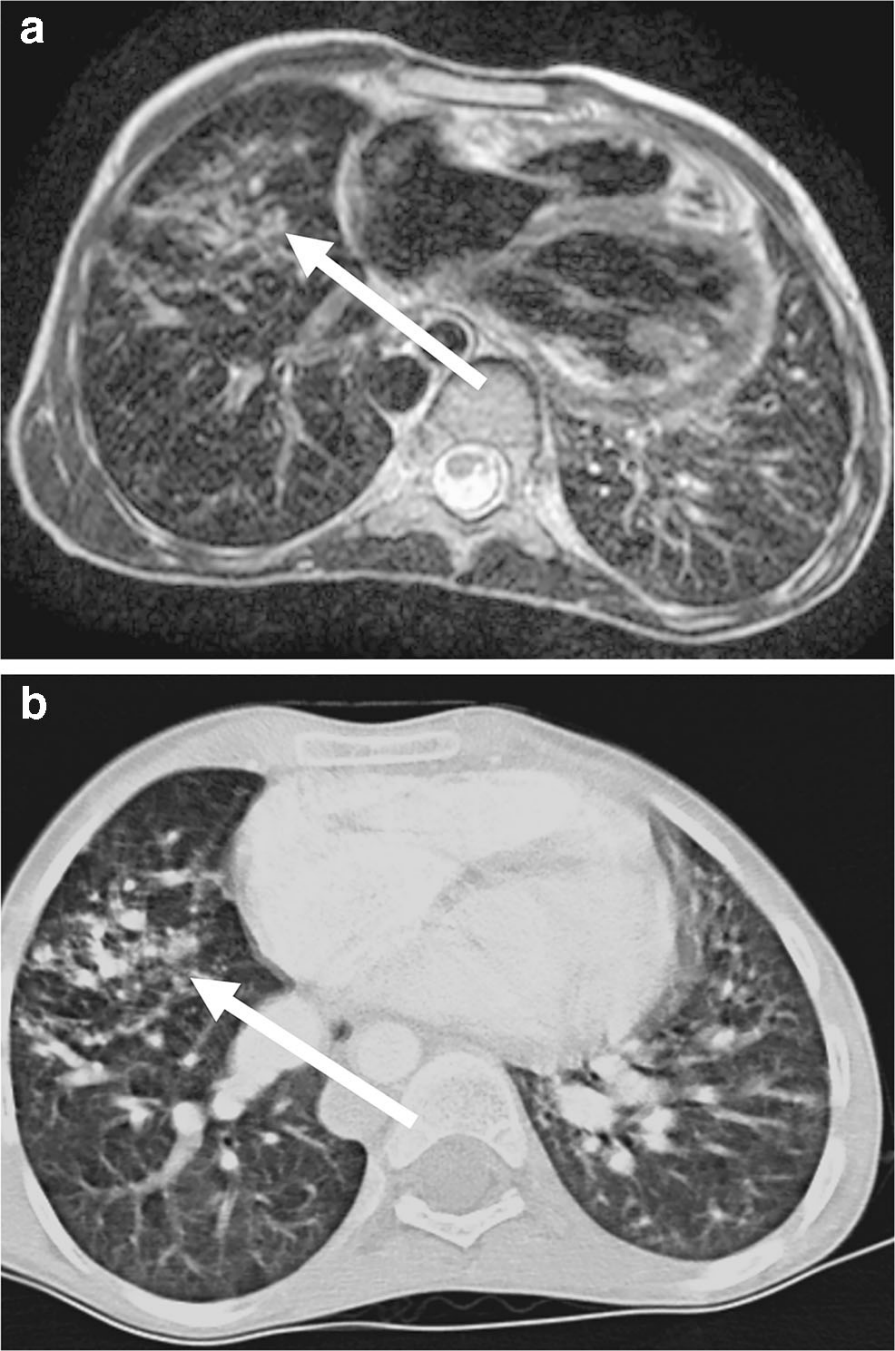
Pulmonary vascular malformation in a 9-year-old boy. a Axial T2-weighted turbo spin-echo MR (parameters in Table 2) shows multiple arteriovenous shunts (*arrow*). b Identical representation (*arrow*) in matching axial contrast-enhanced CT
